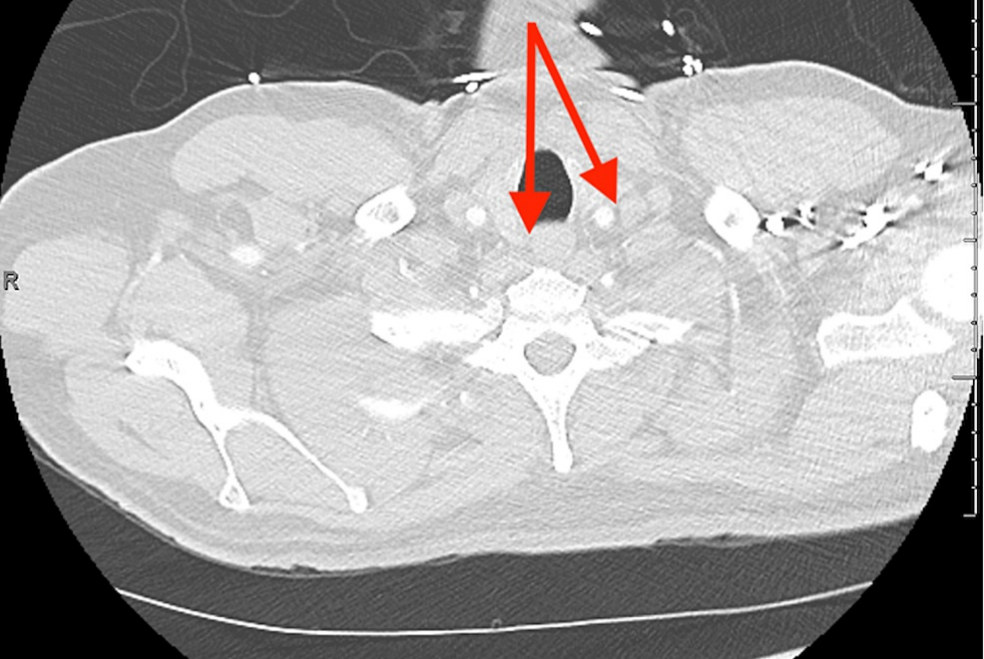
New lymphadenopathy on CT-PE
